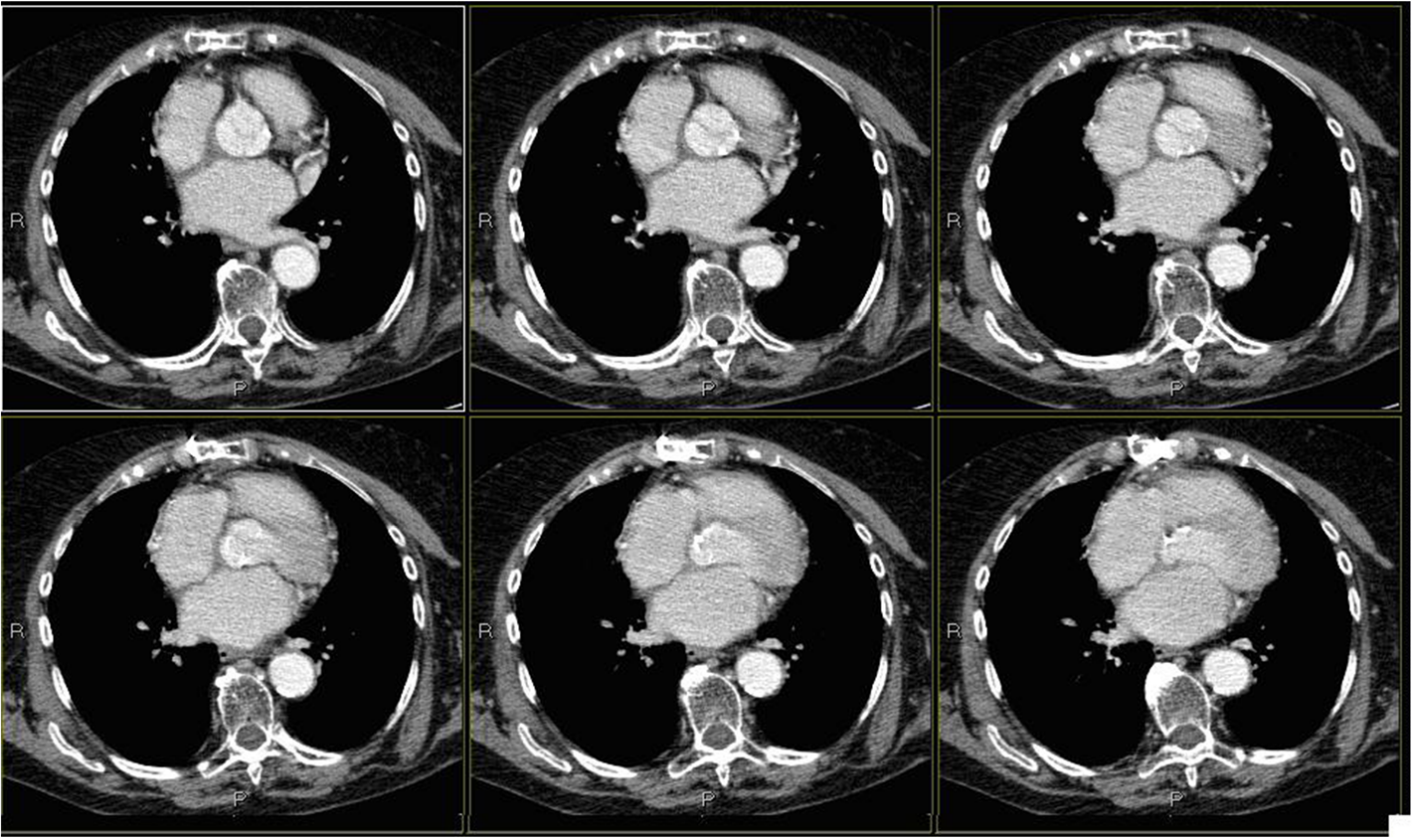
Computed tomography of the chest performed one year after the end of radiotherapy. Image describes total disappearance of the lesion.
